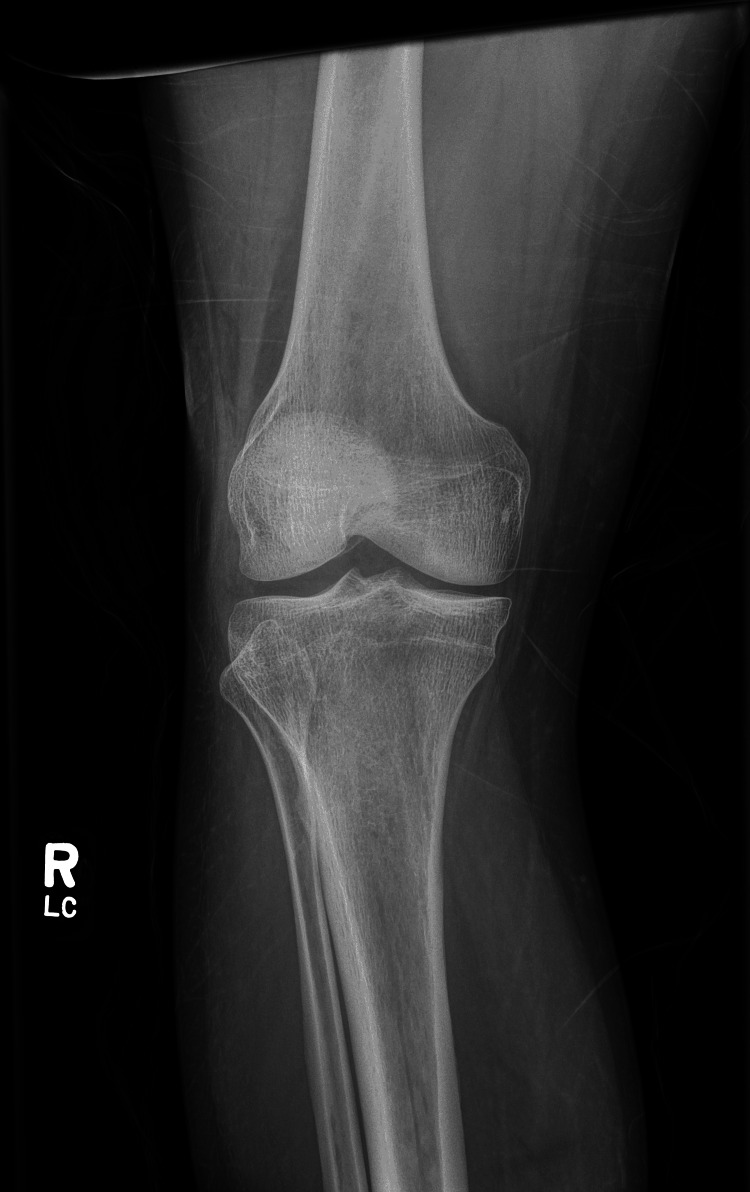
Anteroposterior (AP) x-ray of the right knee did not reveal any fractures or dislocations.
